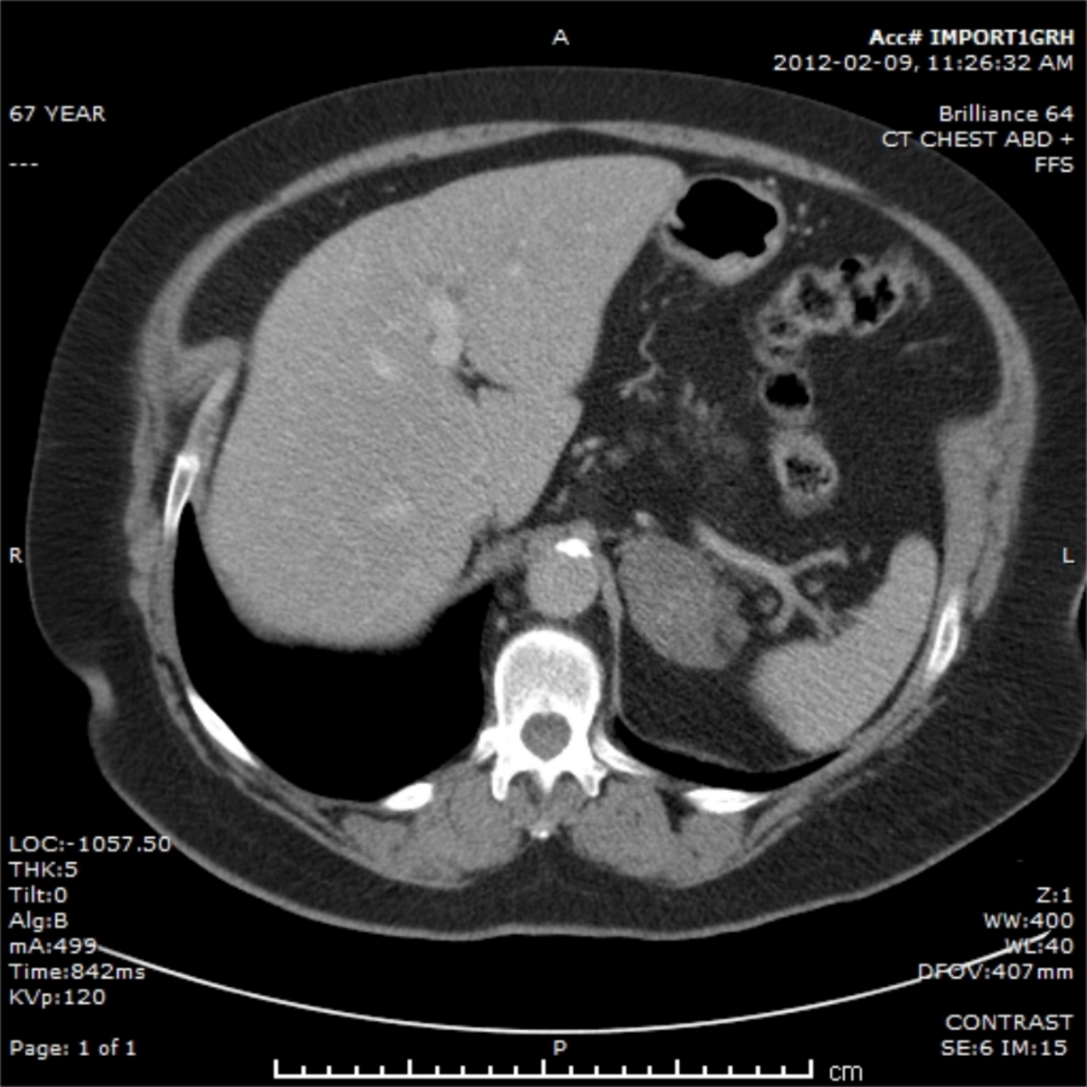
Initial computed tomography (CT) scan of the adrenal oligometastasis.
